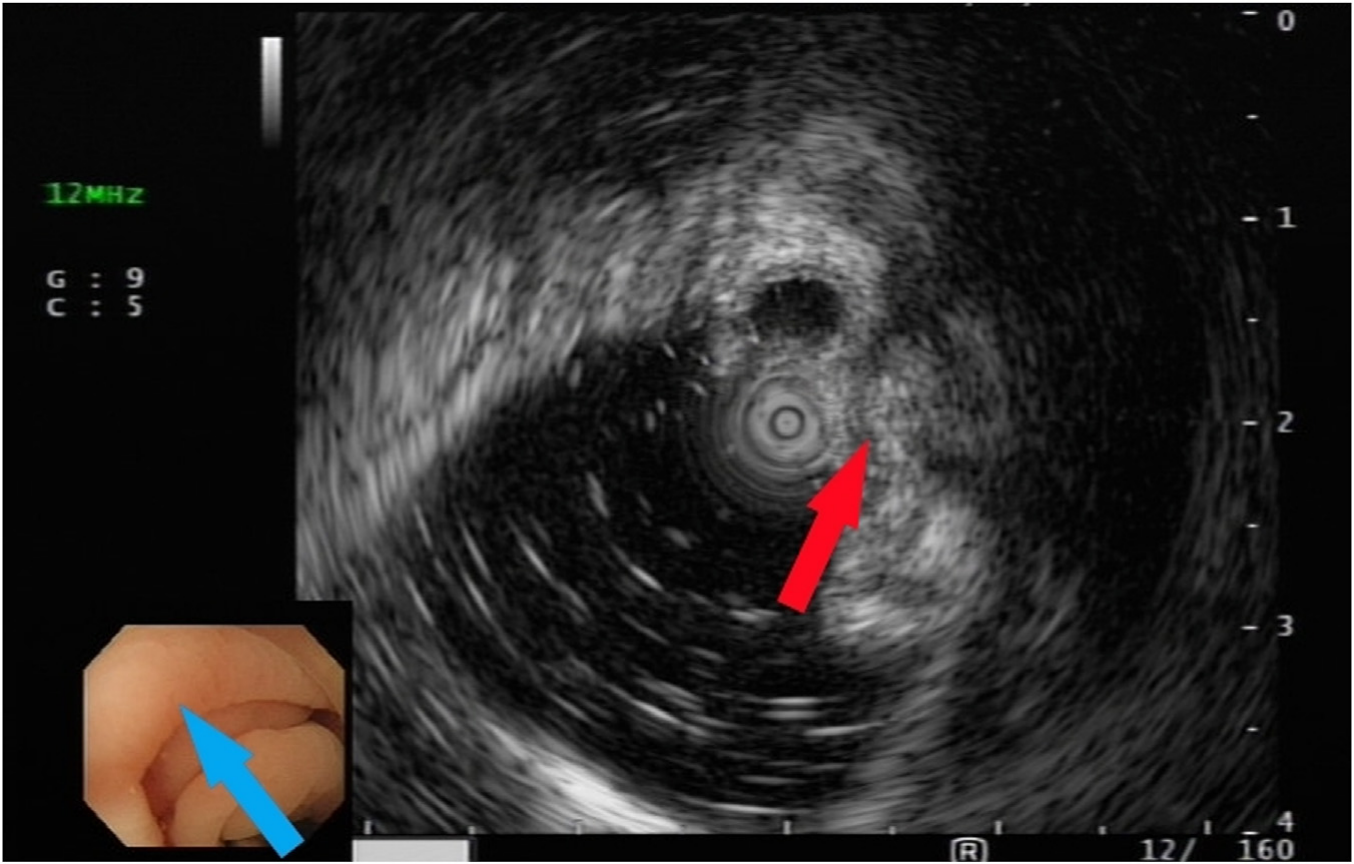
Endoscopic ultrasound image identifying the orientation of the appendiceal lumen relative to the appendiceal cavity (*red arrow*) and guiding the corresponding direction for appendicoscopic intubation (*blue arrow*).
